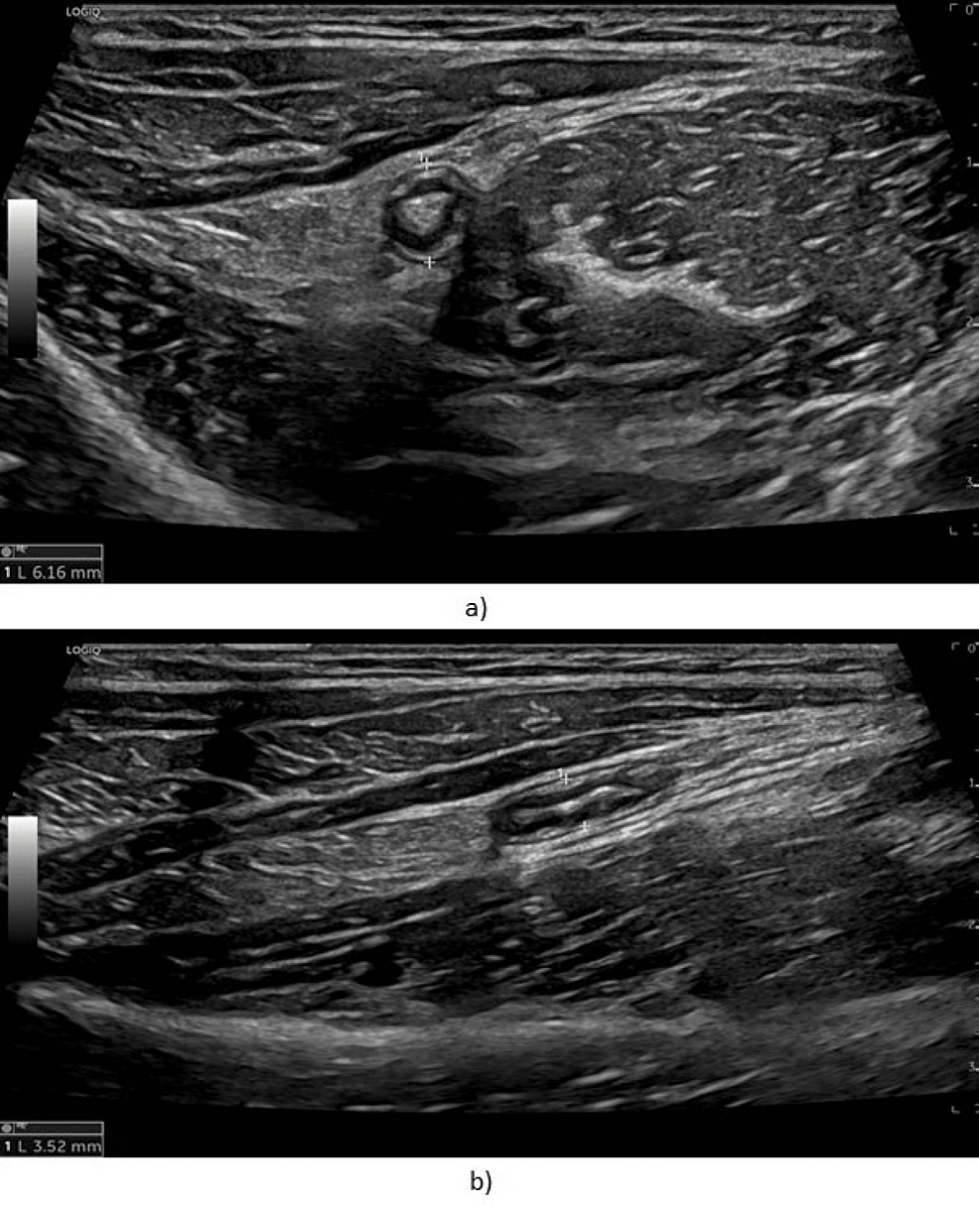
Ultrasound examination showing an appendix with a diameter of 6-7 mm associated with minimal parietal thickening and discrete hyperechogenicity of the adjacent adipose planes (limiting crosses).
